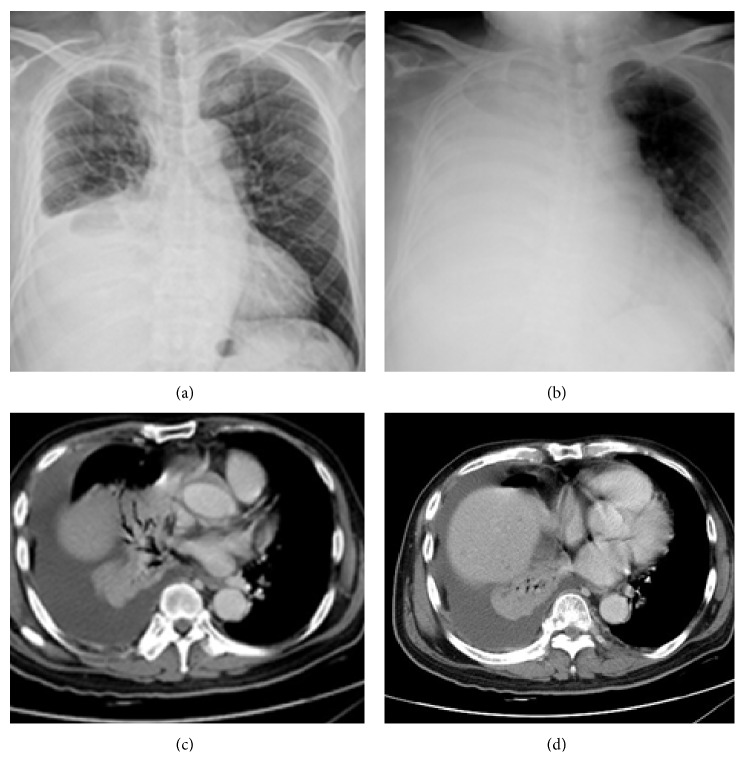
Pulmonary vein obstructive syndrome (PVOS). A 61-year-old man with lung cancer. Chest X-ray (a) before and (b) at the onset of PVOS showed right low lung increase haziness to total opacity. CT scan ((c) and (d)) revealed lung tumor and atelectatic lesions surrounding the superior and inferior pulmonary veins with right pleural effusion.
